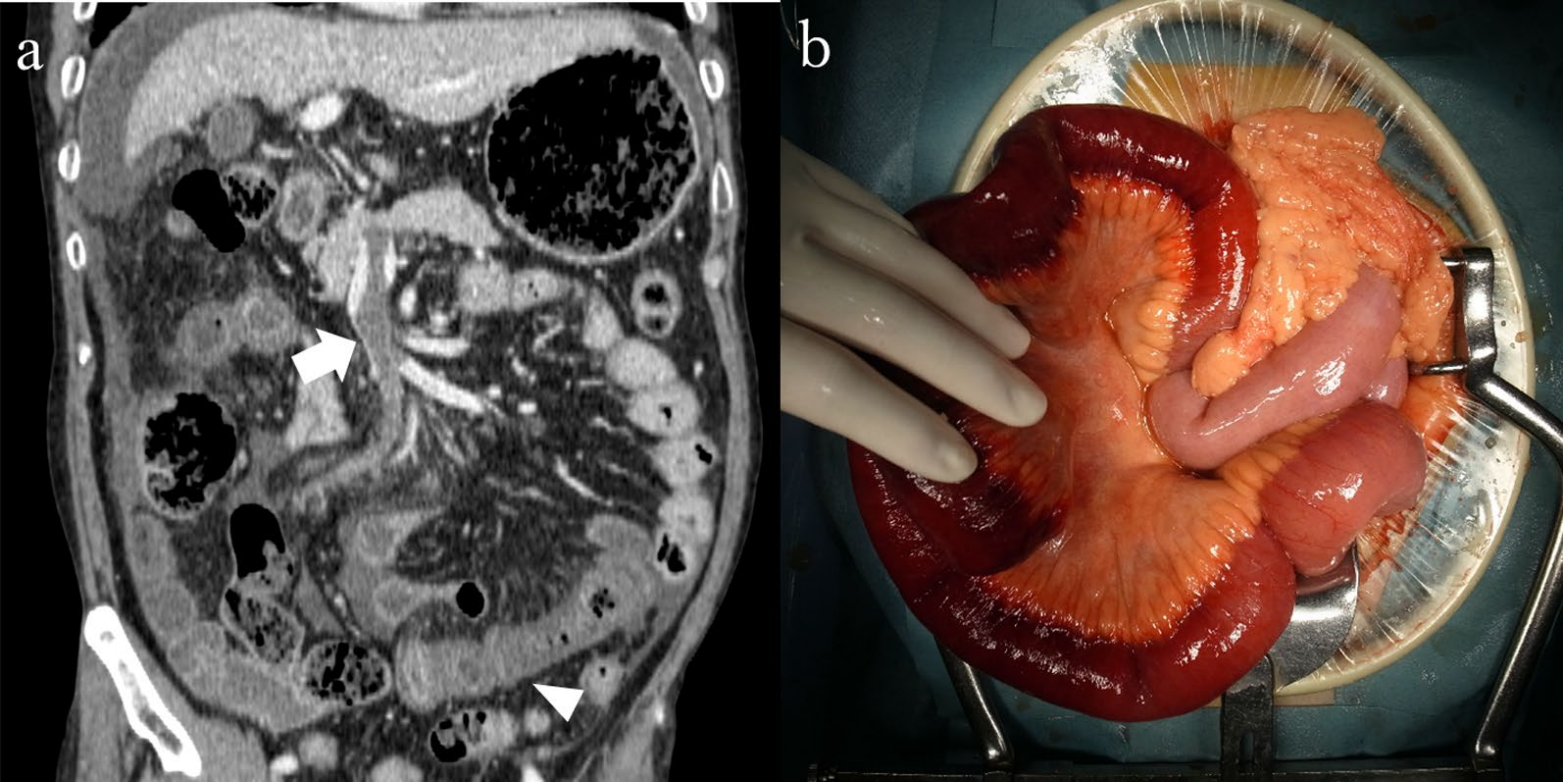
a CE-CT findings (coronal view) of Case 3. Thrombus partially occludes SMV (arrow). Small bowel wall with poor contrast enhancement and massive ascites are observed (arrowhead). b Intraoperative findings. A gangrenous small bowel measuring 65 cm is resected
